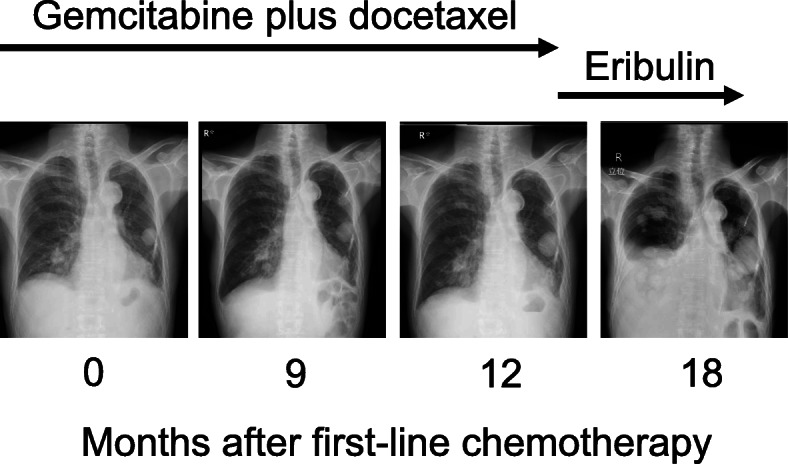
Serial chest radiographic findings after chemotherapy
